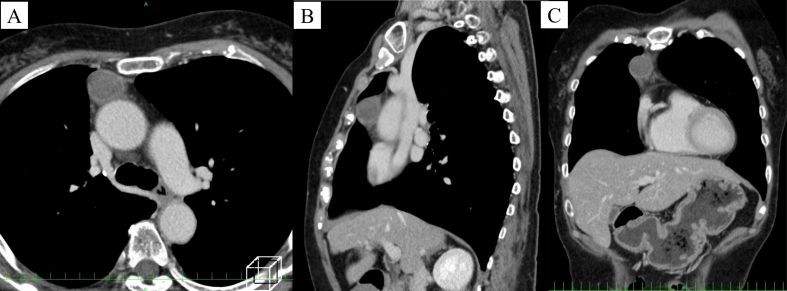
(A) Contrast-enhanced computed tomography showing a nodule with low attenuation in the center and enhancement at the periphery. (B) The sagittal view. (C) The coronal view.
